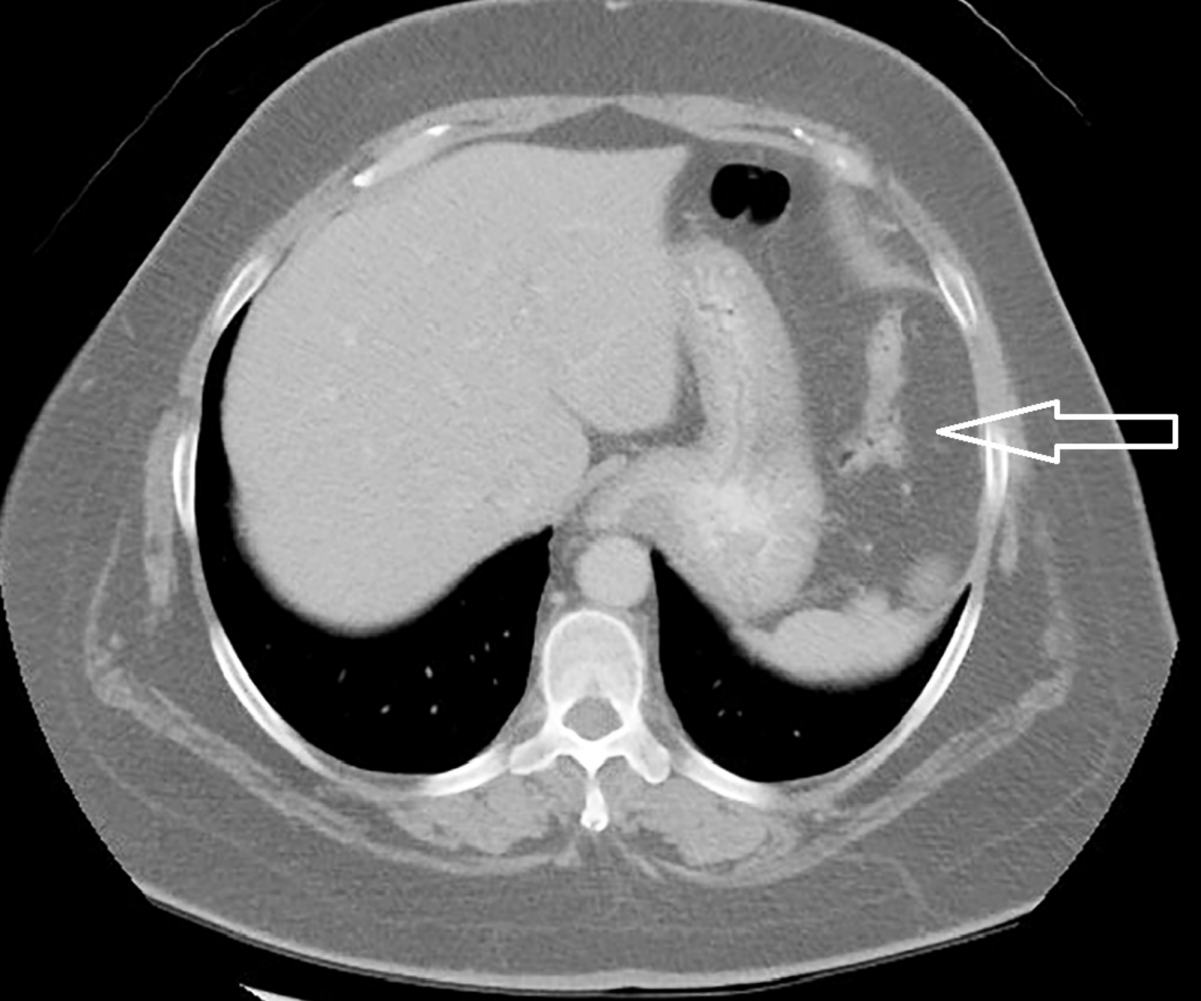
Computed tomography of the abdomen showing a wedge-shaped infarct area measuring 39 mm x 28 mm x 50 mm in the anterior aspect of the spleen (white arrow).
